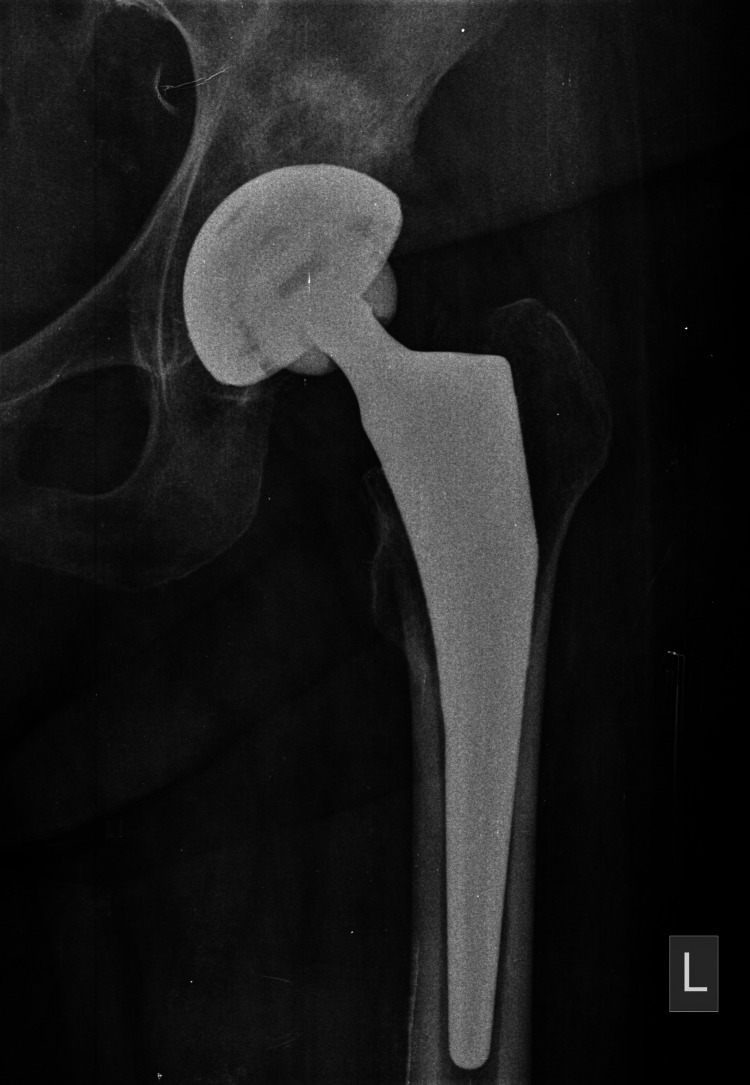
Post-operative x-ray of the Profemur® TL standard stem
